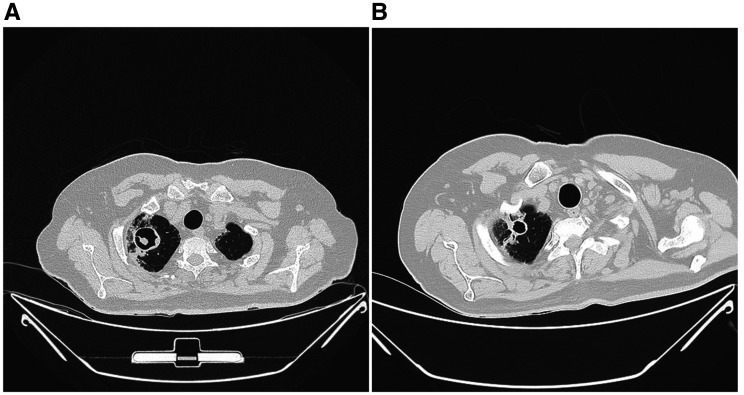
(A) High-resolution computed tomography (HRCT) thorax showing an increase in the size of the cavitatory lesion as well as the opacity inside it. (B) HRCT thorax showing the decreasing size of the cavitatory lesion with a resolved inner opacity.
